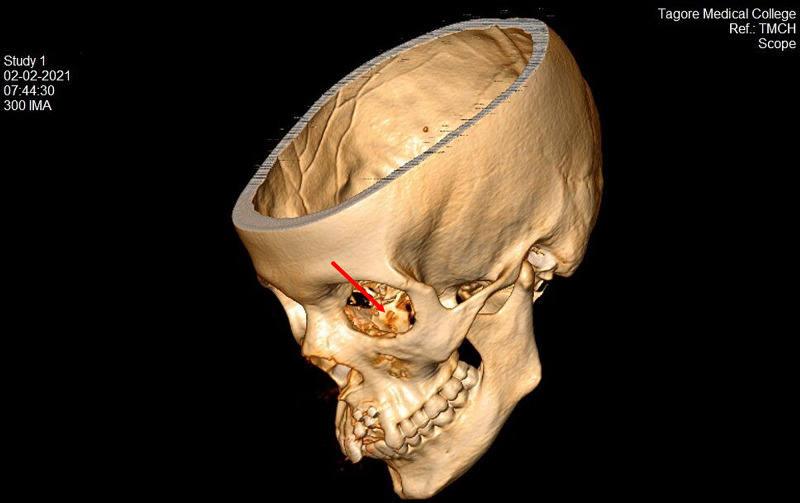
Postop 22-month CT scan (3D) view showing the graft in position (red arrow) in the left orbit
